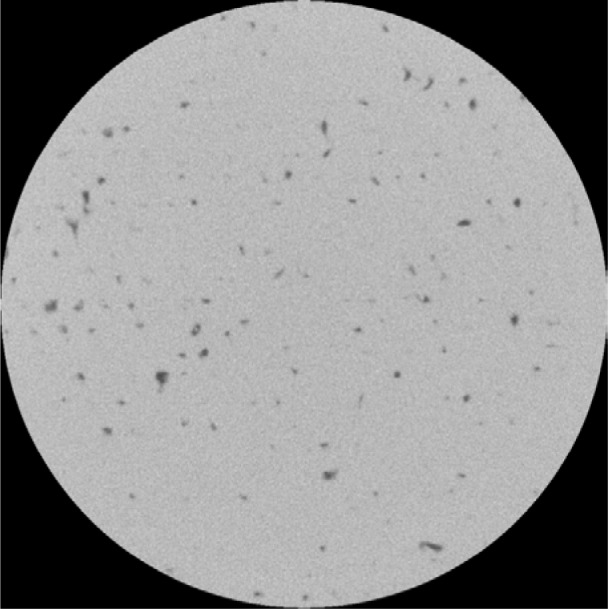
Sample 1–2D, X-ray CT of the interior of a 5 mm diameter cylinder cut from the original sample. The 5 mm cylinder had the same height as the original sample, but the image stack only extends part way through the sample. The pixel size in this image is 2.54 µm. Table 7 gives more details about the images taken from this sample.
